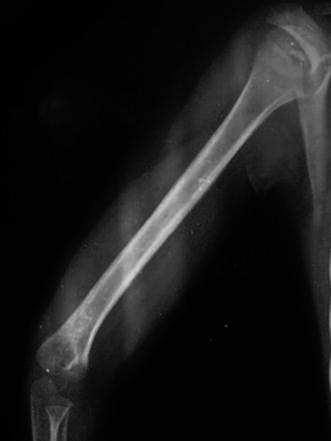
Roentgenogram of the right humerus showing a diffuse periosteal reaction with a permeative type of destruction involving almost the whole of the diaphysis and a huge soft-tissue shadow surrounding the diaphysis
